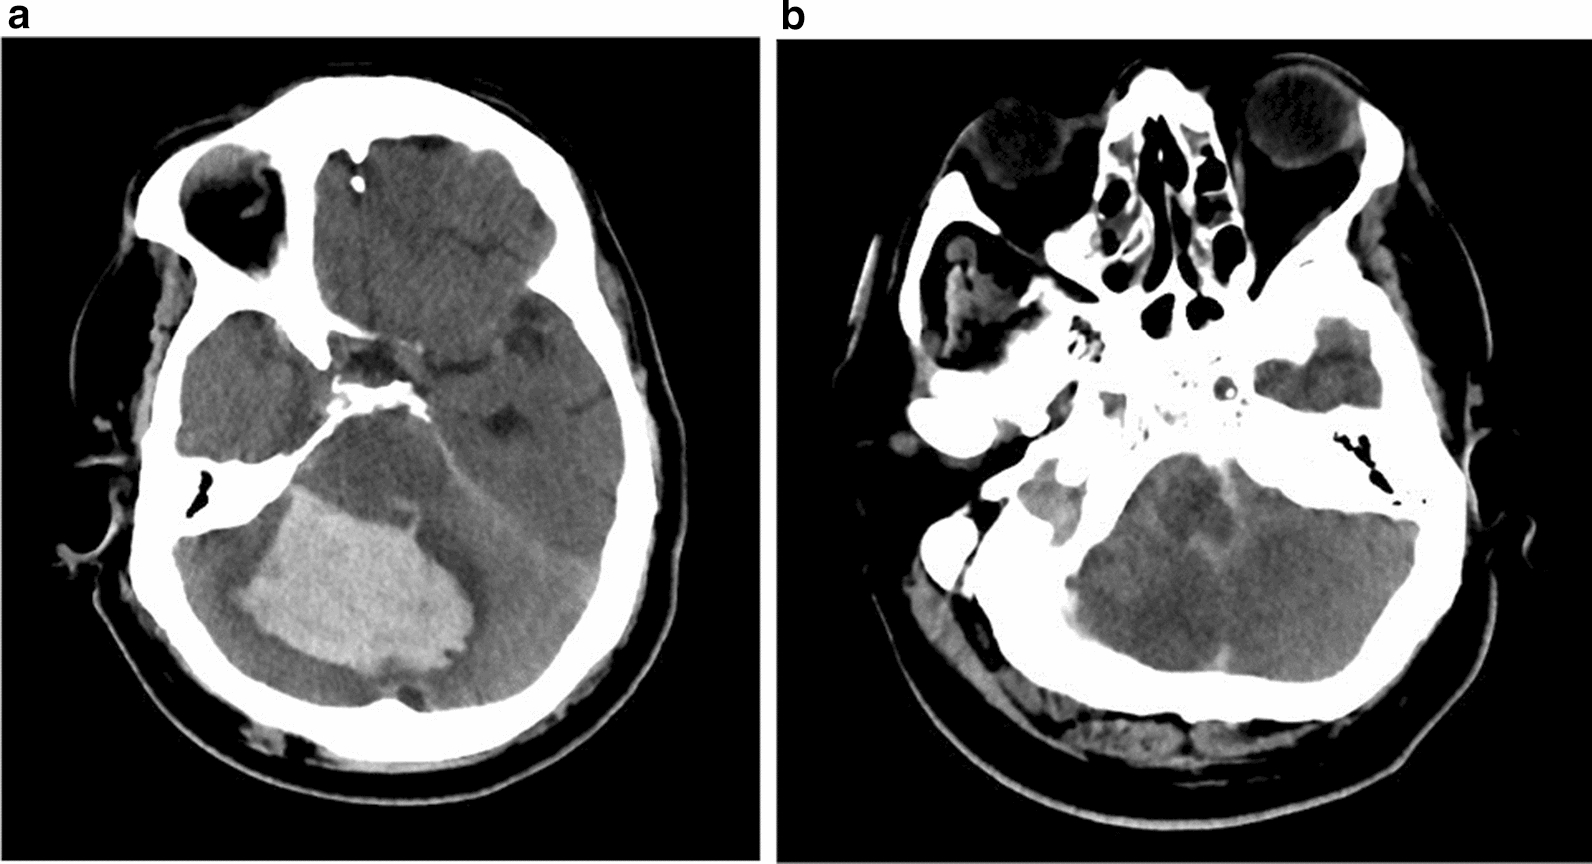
Axial non-contrasted head CT scans of the posterior fossa. The images show a large cerebellar hematoma with surrounding edema (a) as well as edema in the medulla (b)
